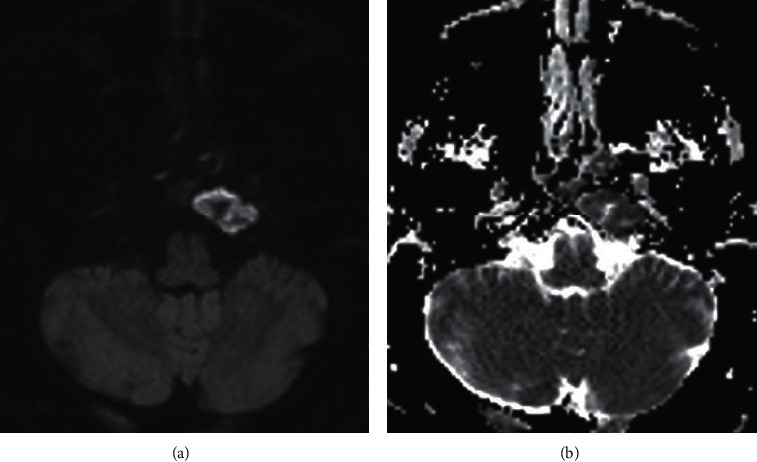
(a) Axial diffusion-weighted image (DWI). (b) Apparent diffusion coefficient image (ADC): heterogeneously restricted diffusion (increased signal on DWI and decreased signal on ADC) in the petrous apex lesion, likely reflecting viscous fluid. Simple cholesterol granulomas typically show decreased DWI signal.
